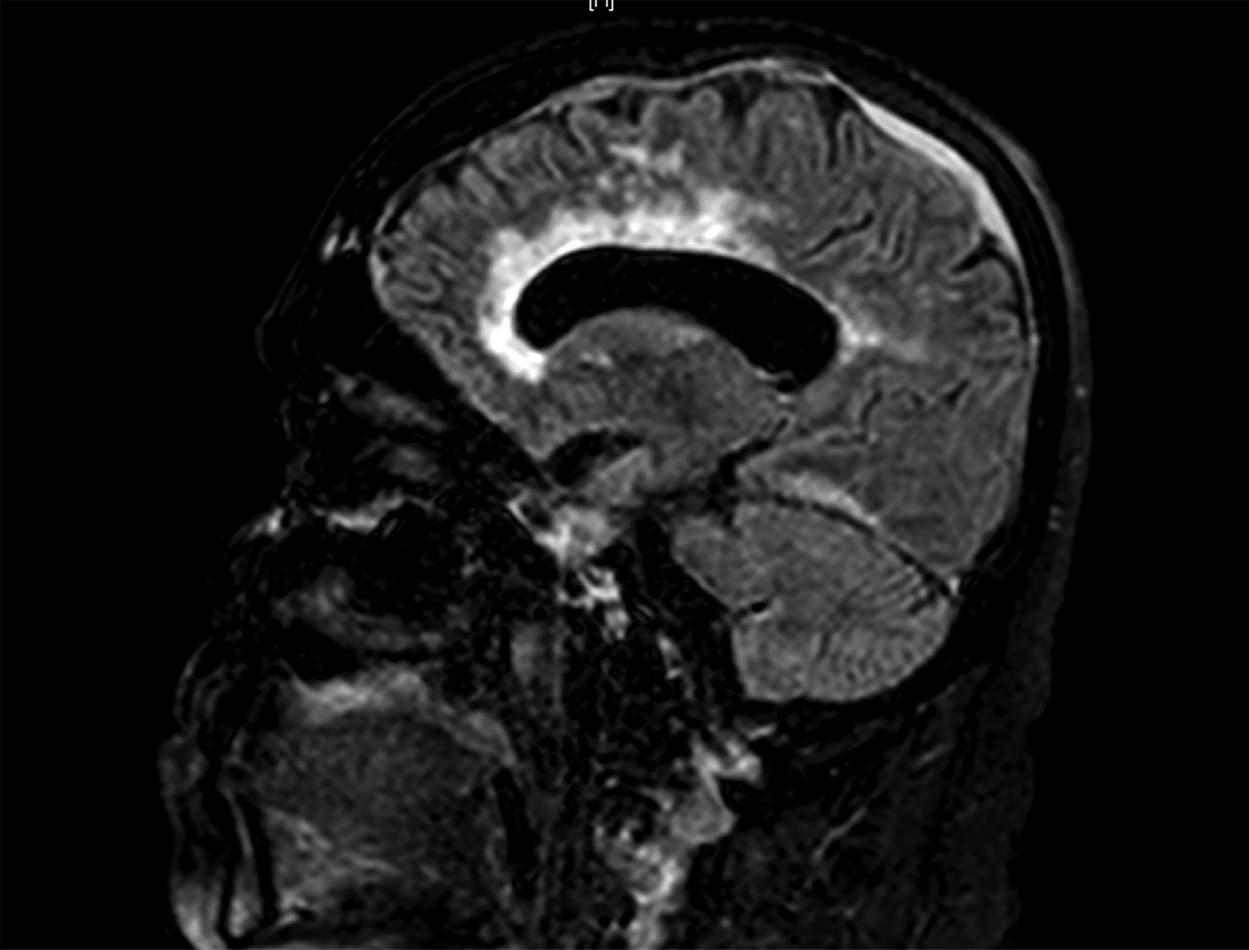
Magnetic resonance imaging revealing diffuse leucoencephalopathy and meningeal enhancement (occipital predominance).
